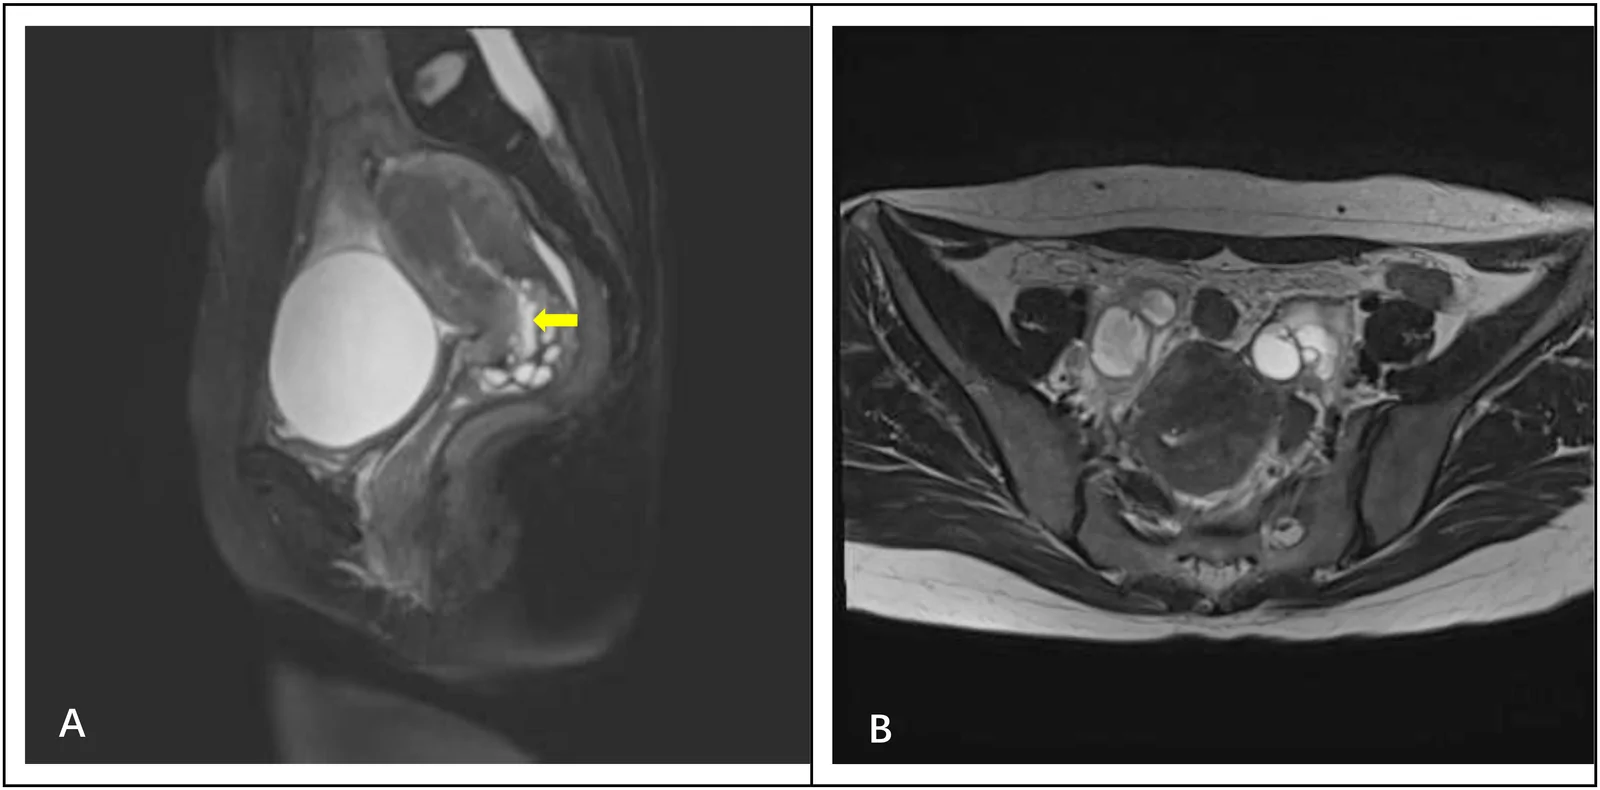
Imaging findings. (A) Pelvic MRI T2WI suggested a soft tissue mass and multiple small cysts of the cervix (yellow arrow). (B) Both ovaries were multilocular cystic with high signal on T2WI.
